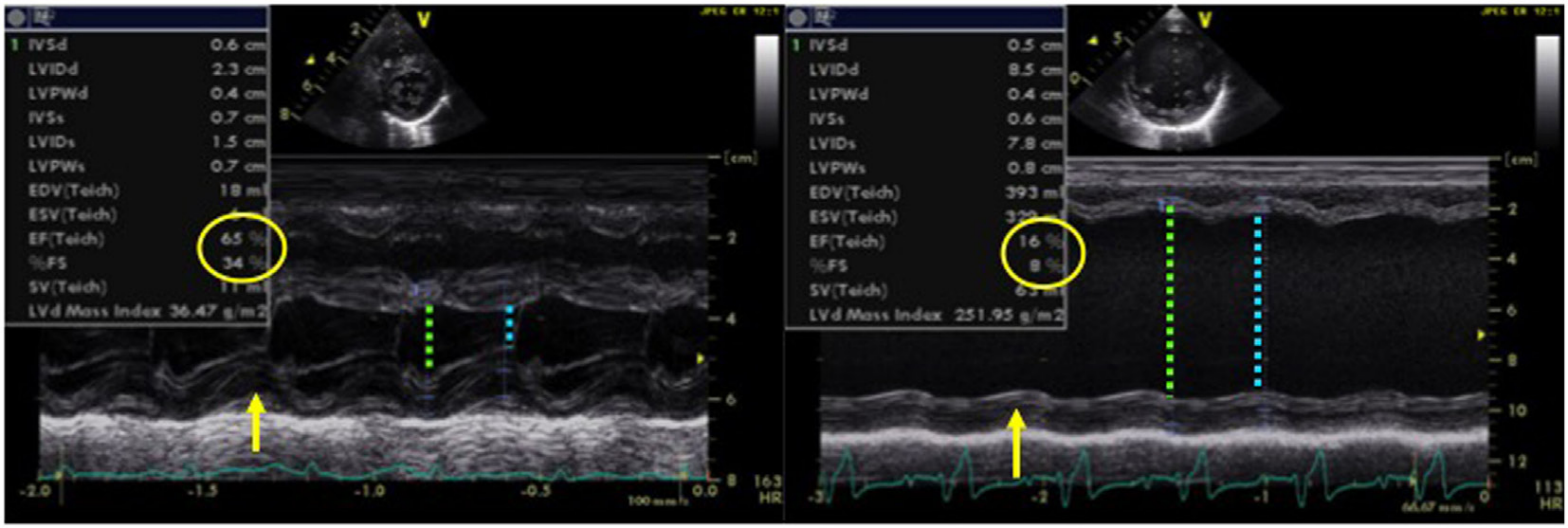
M-mode echocardiography obtained from a patient (left image) with normal left ventricular systolic function (FS = 34% and EF = 65%) and in a patient (right image) with dilated cardiomyopathy with severe left ventricular systolic dysfunction (FS = 8% and EF = 16%), LVEDD with green arrow and LVESD with blue arrow. Abbreviations: EF, ejection fraction; FS, fractional shortening; LVEDD, left ventricle end-diastolic dimension; LVESD, left ventricle end-systolic dimension.
